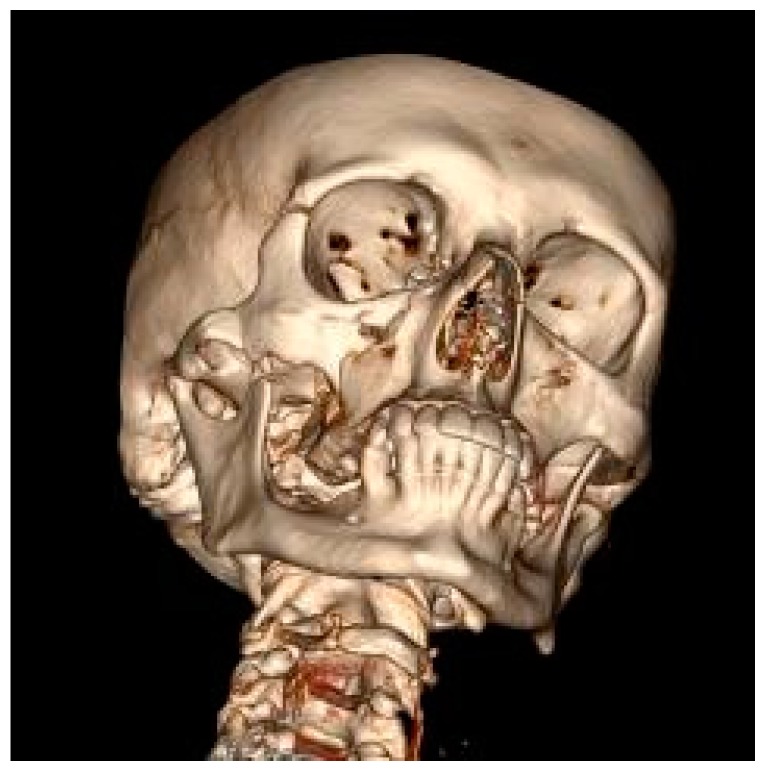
Right zygomatic bone fracture (computed tomography with 3D reconstruction).
